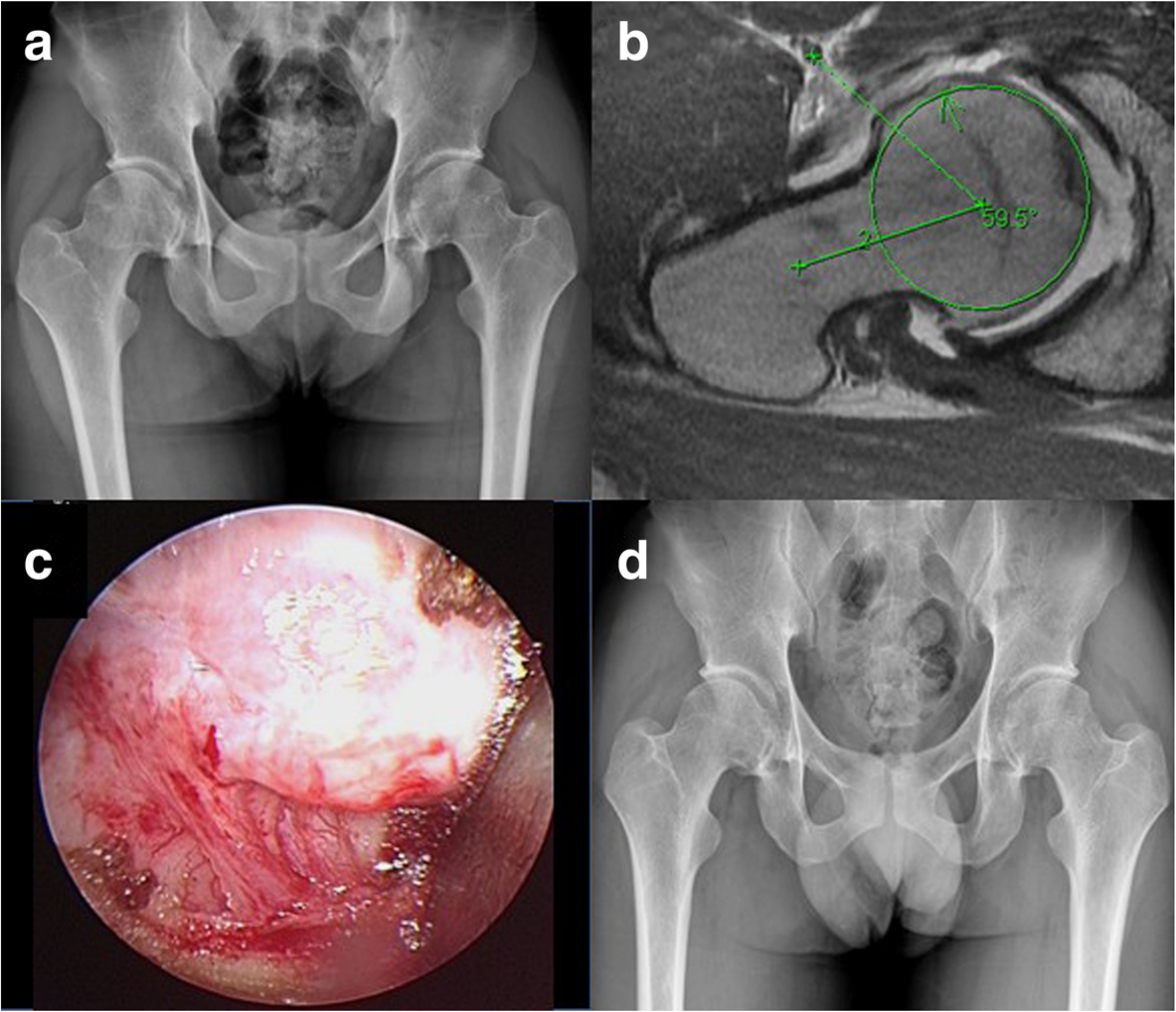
A 23-year-old male patient with bilateral proximal femur cam-type FAI. a Preoperative pelvis radiograph. b Preoperative right hip MRI axial view. c intraoperative arthroscopic image showing the bony bump. d Follow-up radiography of pelvis AP view at postoperative 3 years
